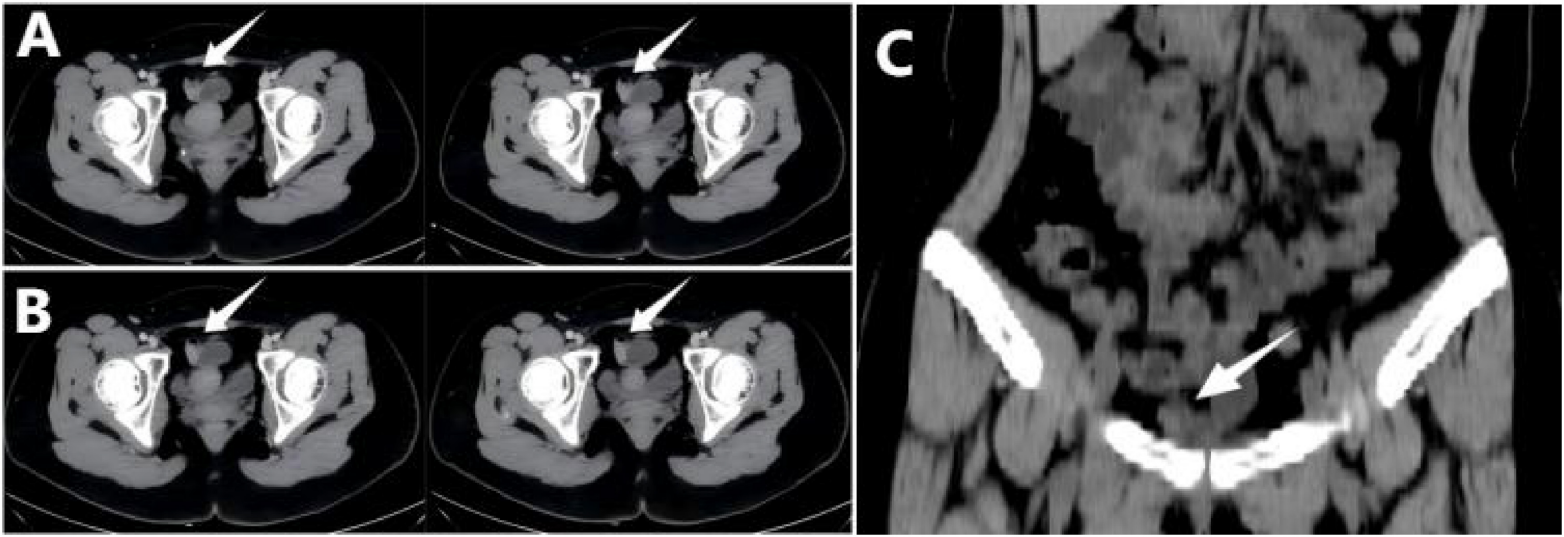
Multi-slice CT imaging of the small intestine. (A,B) Axial views show a single nodular hyperdense lesion with relatively well-defined borders, indicated by white arrows. (C) The coronal view demonstrates the corresponding hyperdense lesion, indicated by a white arrow.
